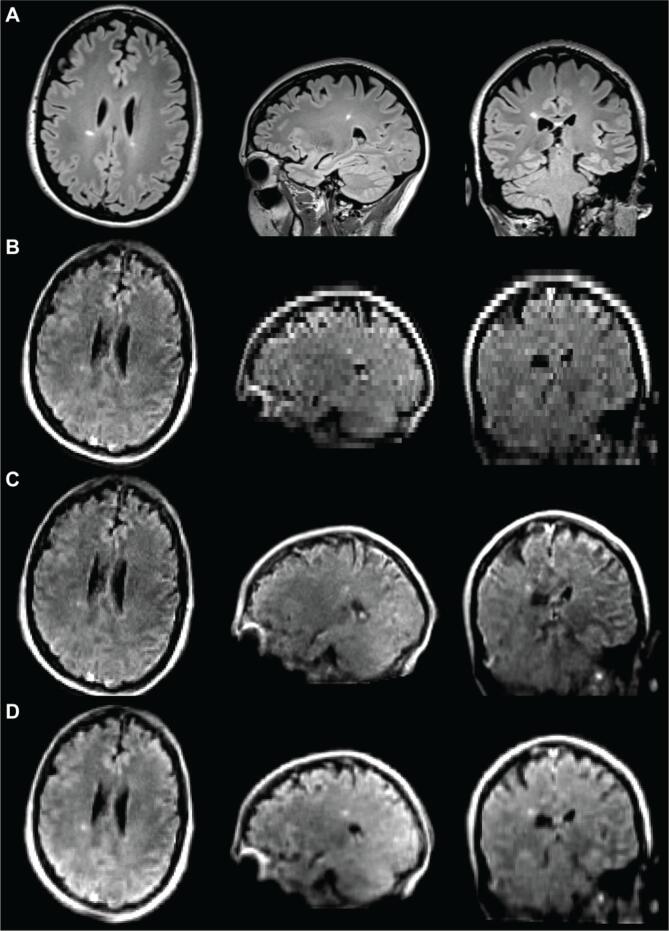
Super-resolution images generated from orthogonal slice directions. This figure demonstrates a super-resolution approach using anisotropic image acquired in orthogonal slice directions (axial, sagittal, and coronal). The patient is a 69-year-old man with stable RRMS. (A) 3T FLAIR imaging demonstrates a right periventricular lesion (conspicuity = 0.18) in the three orthogonal planes. (B) 64mT imaging of the same lesion (conspicuity = 0.11) using an axial FLAIR acquisition with sagittal and coronal reformatted images. (C) Corresponding axial, sagittal, and coronal slices from separate acquisitions in each direction (conspicuity: axial = 0.11, sagittal = 0.09, and coronal = 0.13) registered to the axial image by affine transformation. (D) Corresponding super-resolution images generated by averaging the coregistered axial, sagittal, and coronal acquisitions in C (conspicuity: linear = 0.11, nearest-neighbor = 0.13).
